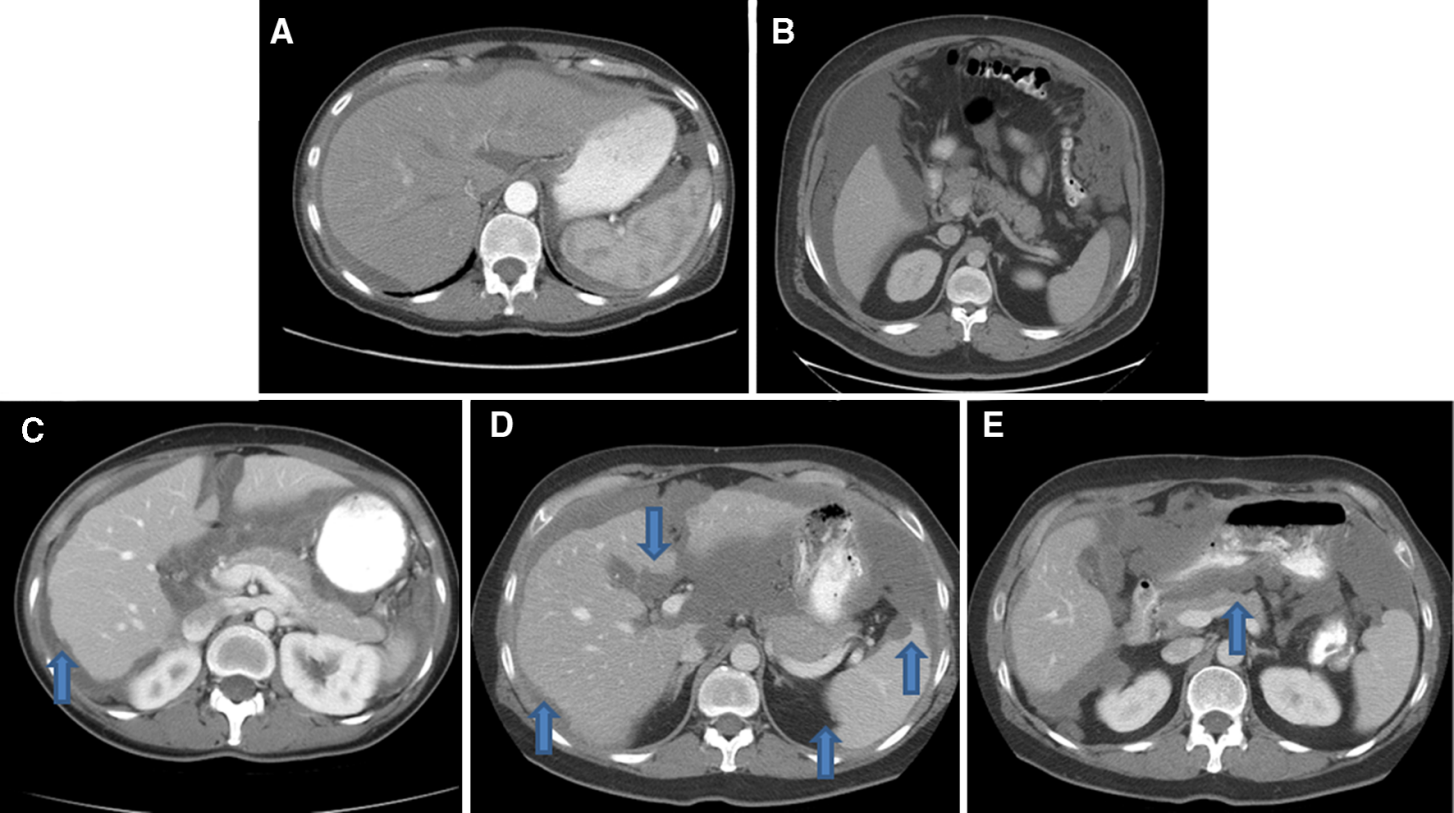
SPAAT scoring of visceral organs. a Mucinous ascites around the liver and spleen; however, the border is smooth without evidence of scalloping and this would be assigned zero points. b Mucinous ascites with a smooth spleen and pancreas border. Again, zero points are assigned. c CT scan findings of scalloping of the liver (*arrow*). d Liver and spleen scalloping (*arrows*). e Loss of the smooth pancreatic border with indentation of the organ (*arrow*), representing a CT finding that would score one point. *SPAAT* simplified preoperative assessment for appendix tumor, *CT* computed tomography
